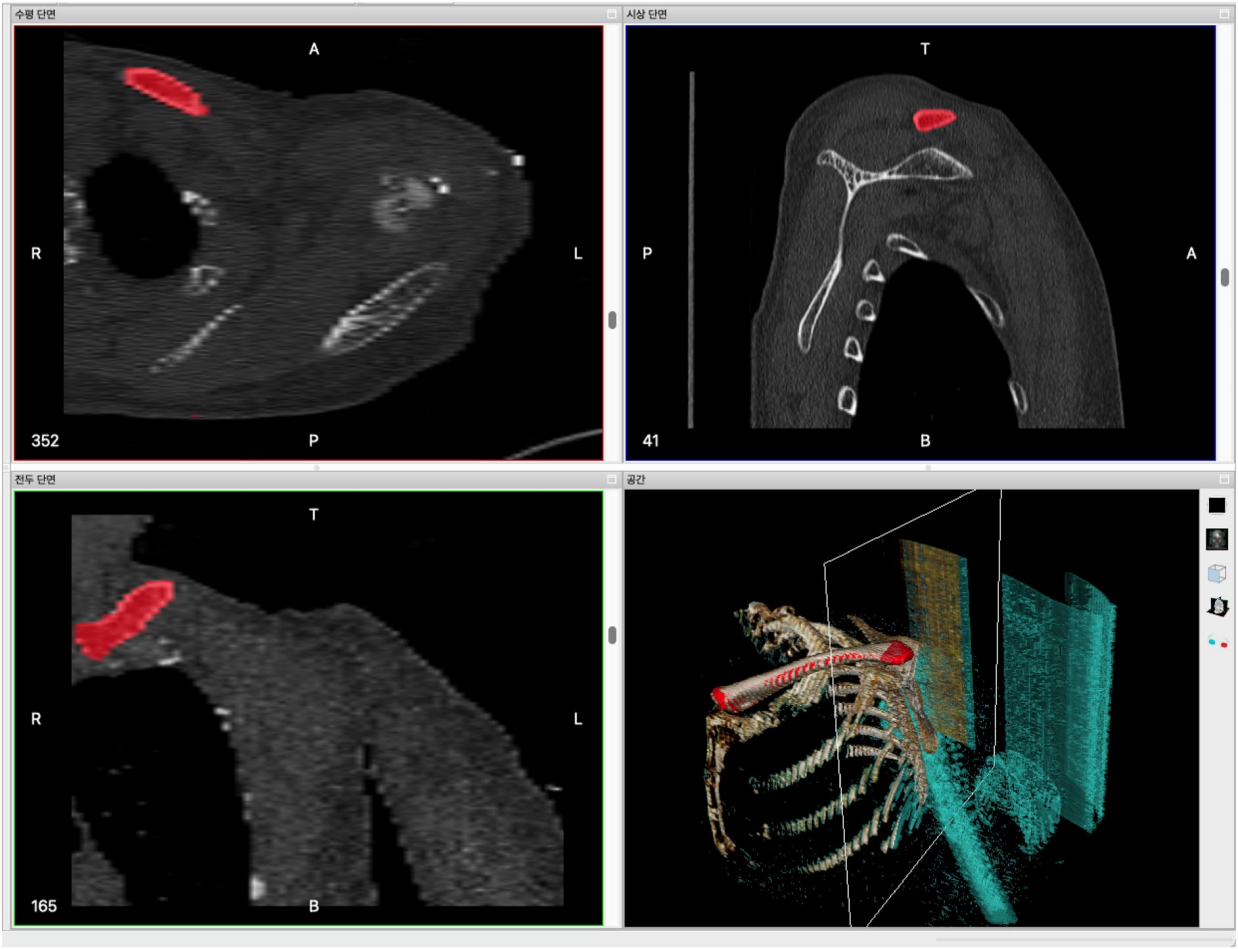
Image of CT(computerized tomography) cross-sectional photos synthesized and converted into a 3D structure using the InVesalius program.
